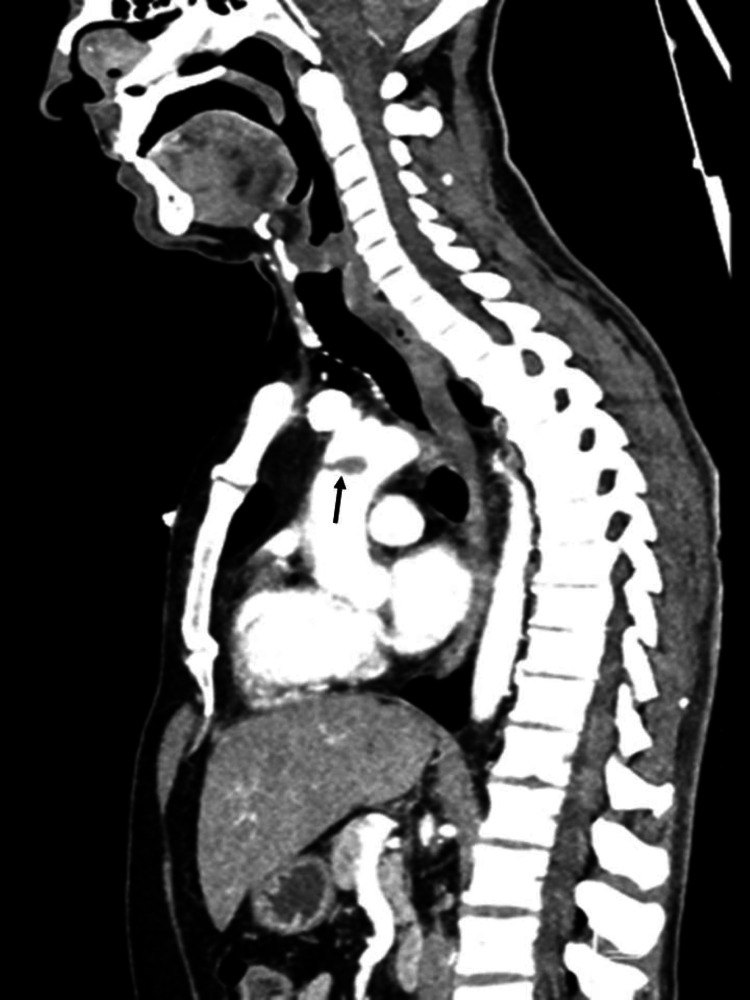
CTA showing an endoluminal repletion defect in the ascending aorta (sagittal view) CTA: computed tomography angiography
